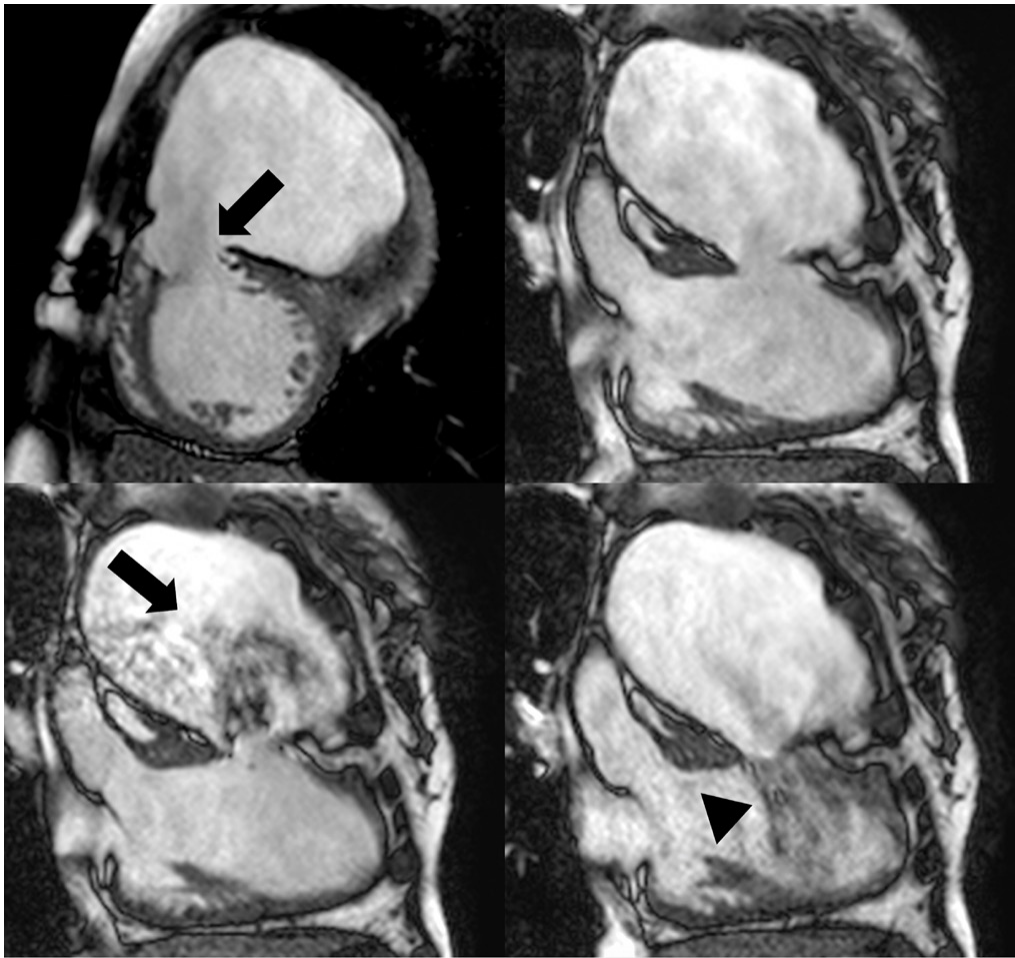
Left ventricular short-axis cinemagnetic resonance image (upper left) showing the mid-anterior wall disruption point (arrow). Oblique left ventricular long-axis cine images during end diastole (upper right), systole (bottom left), and mid diastole (bottom right). Turbulent flow is depicted from the left ventricle to the pseudoaneurysm in the systole (arrow), with reflow during diastole (arrowhead).
